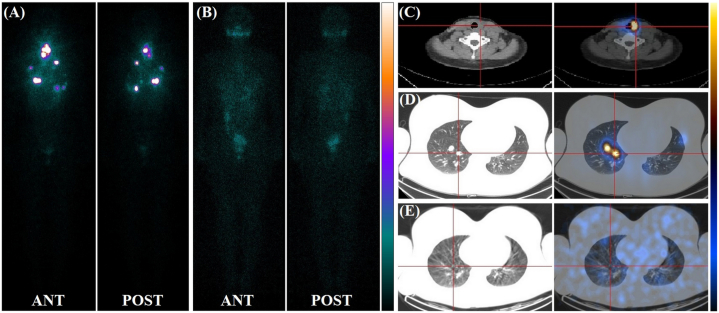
Iodine-131 treatment for DTC pulmonary metastases achieved ER (the Rx-WBS imaging showed focal distribution). Picture specification: A 17-year-old girl had follicular carcinoma of the left lobe of the thyroid with lymph node metastases in area VI (2/4). Chest CT: The maximum diameter of the pulmonary metastases was 1.73 cm. Received twice ^131^I treatments at a activity of 5.55GBq/time. Whole body anterior (ANT) and posterior (POST) plane imaging and SPECT/CT imaging after ^131^I treatment. Imaging after initial ^131^I treatment (A,C,D): Faint ^131^I uptake in the thyroid bed and pulmonary metastases showing focal ^131^I uptake. Imaging after the second ^131^I treatment (B,E): The residual thyroid gland and pulmonary metastases were completely cleared; TgAb negative; sTg value before initial ^131^I treatment was 3314 ng/mL, TSH-suppressed Tg value was＜0.04 ng/mL after the 2nd ^131^I treatment.
